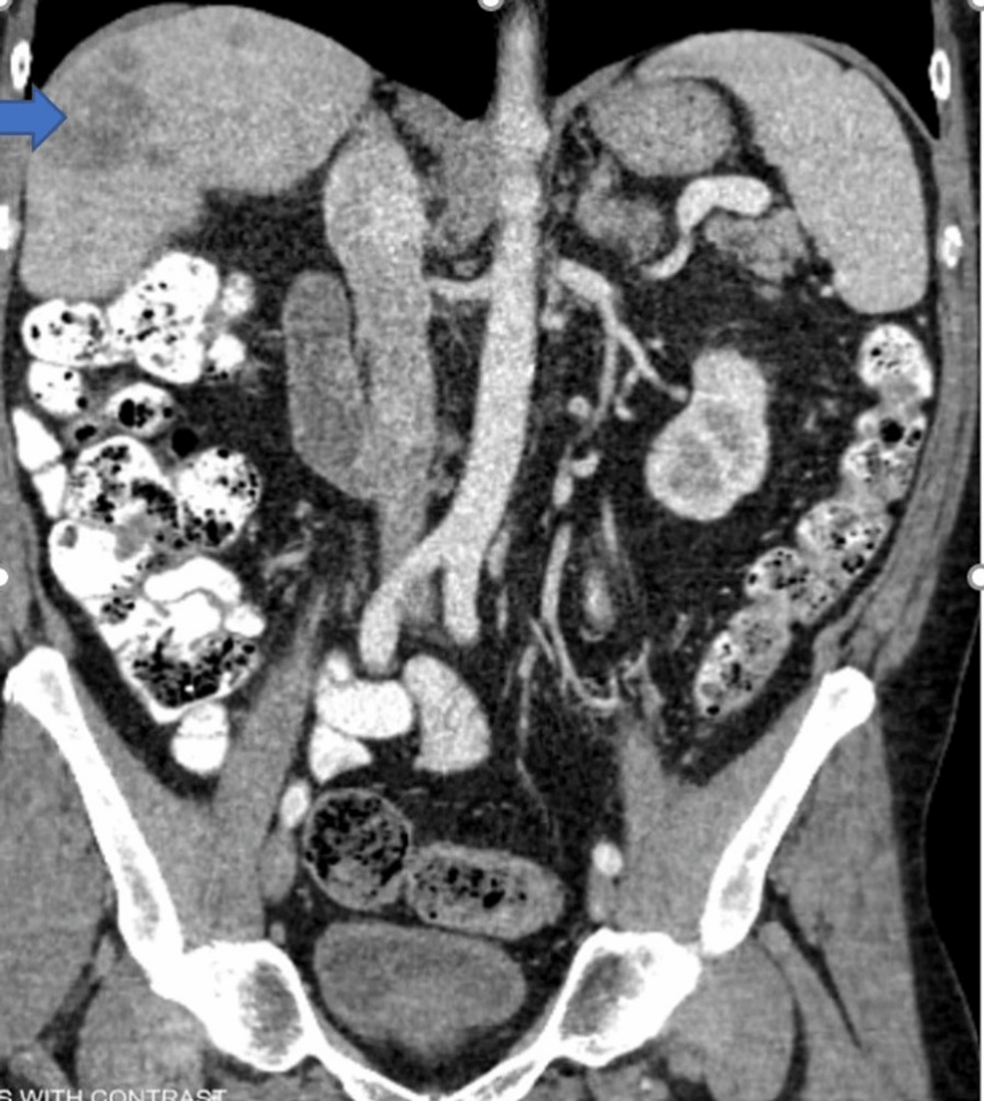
Contrast-enhanced CT of the abdomen and pelvis, coronal view with liver metastasis (blue arrow)
